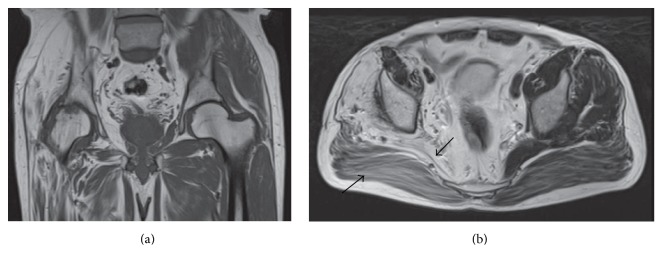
MRI of the hip taken 10 months after the starting of treatment: (a) coronal T1-weighted image showing low signal osteoarthritis change and (a and b) coronal T1-weighted and axial T2-weighted images showing homogeneous fatty signal changes in the gluteus medius muscle and similar appearance even in the other muscles (arrows).
